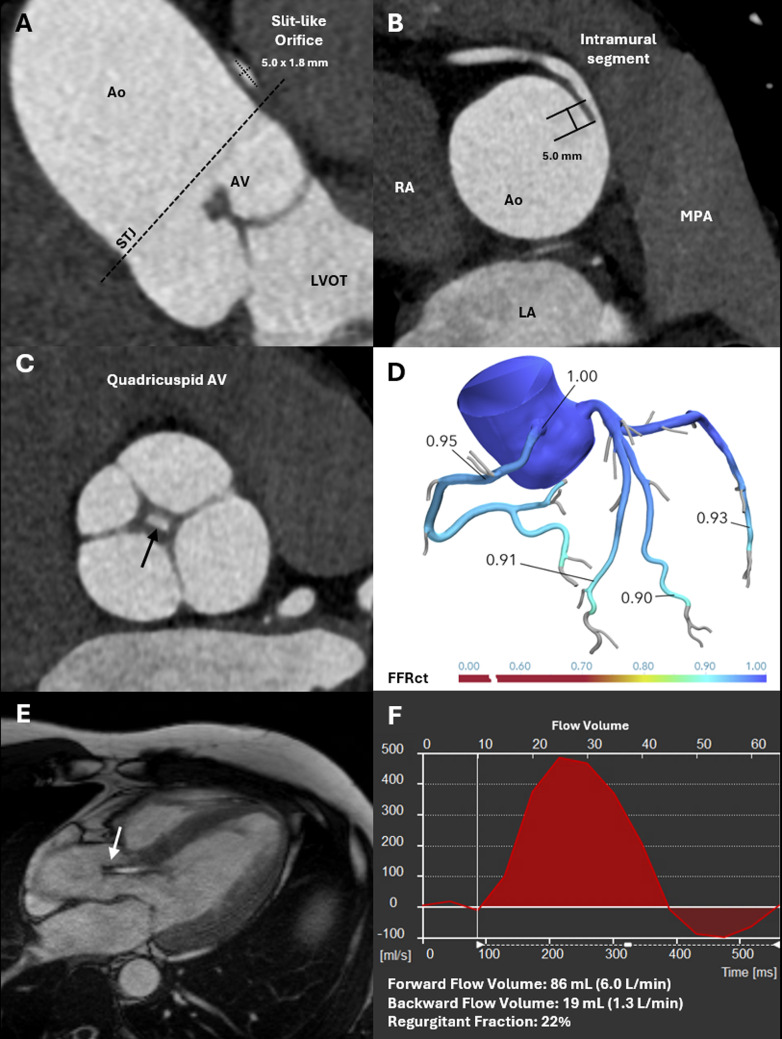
Multimodality imaging of anomalous right coronary artery and quadricuspid aortic valve with aortic regurgitation. (A) Coronary computed tomography angiography, CTA, demonstrating an anomalous right coronary artery, RCA, originating above the sinotubular junction with a slit-like orifice measuring 5.0 × 1.8 mm. (B) CTA showing a 5.0 mm intramural segment of the anomalous RCA within the aortic wall. (C) Short-axis CTA image of a quadricuspid aortic valve with central non-coaptation, black arrow. (D) Computed tomography–derived fractional flow reserve, FFRct, demonstrating preserved coronary physiology along the anomalous RCA, values ranging from 0.90 to 1.00. (E) Cardiac magnetic resonance imaging, three-chamber view, demonstrating an aortic regurgitation jet directed into the left ventricular outflow tract, white arrow. (F) Phase-contrast cardiac magnetic resonance imaging obtained above the aortic valve demonstrating mild to moderate aortic regurgitation with a regurgitant volume of 19 mL and regurgitant fraction of 22%. Abbreviations: Ao, aorta, AV, aortic valve, CTA, computed tomography angiography, FFRct, computed tomography–derived fractional flow reserve, LA, left atrium, LVOT, left ventricular outflow tract, MPA, main pulmonary artery, RA, right atrium, RCA, right coronary artery, RF, regurgitant fraction, STJ, sinotubular junction
